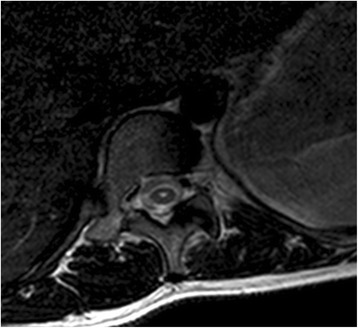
Image demonstrating typical appearance of a persistent central canal on T2-weighted axial MRI of a thoracic spine segment
